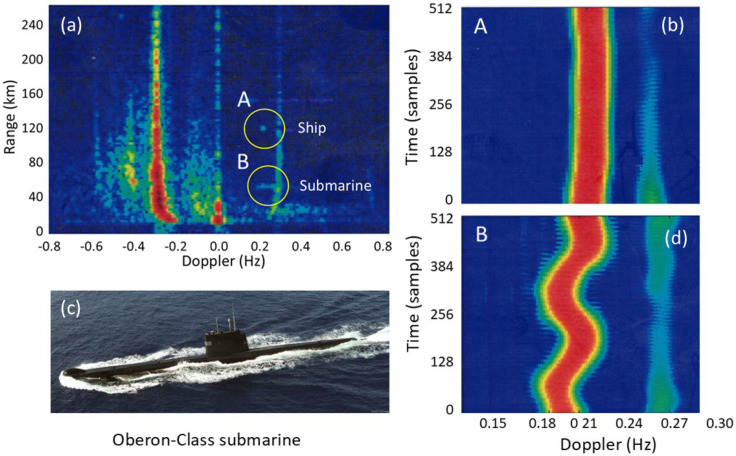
HF echo from a submarine steaming on the surface in a following swell. (a) ship and submarine echoes identified on range–Doppler map; (b) Oberon-Class submarine; (c) time–frequency analysis of ship echo; (d) time–frequency analysis of submarine echo showing modulation.
